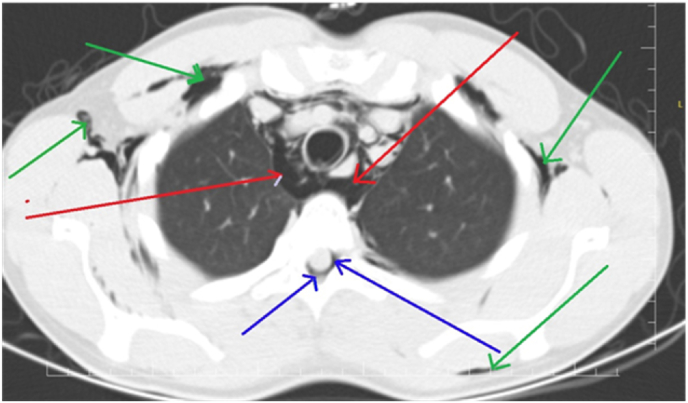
CT scan showing pneumomediastinum (red arrows), pneumorrhachis (blue arrows) and subcutaneous emphysema (green arrows). (For interpretation of the references to colour in this figure legend, the reader is referred to the Web version of this article.)
